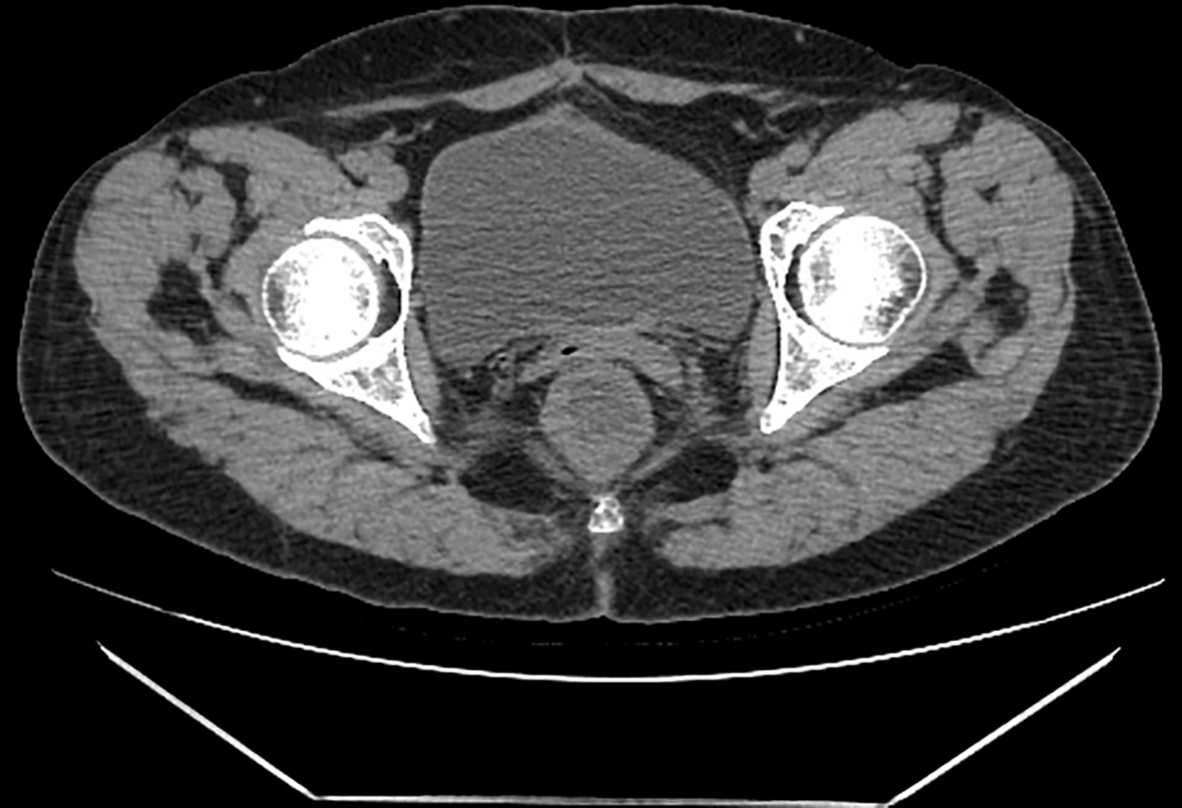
Postoperative CT findings. The patient was reexamined by pelvic CT five years after surgery, and no recurrence or metastasiswas found in the pelvic cavity.
